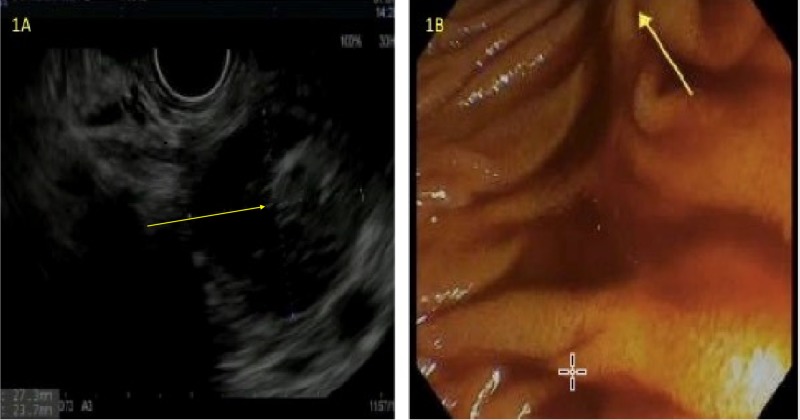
Endoscopic images (A. EUS, B. Upper esophagogastroduodenoscopy) (A) Endoscopic ultrasound (EUS) demonstrating complex pancreatic collection containing hyperchogenic blood-like material. (B) Endoscopic confirmation of bleeding from the ampulla.
